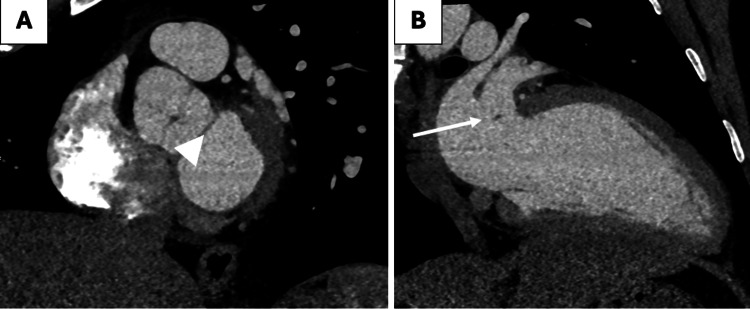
Cardiac CT in short axis (A) and two-chamber (B) planes post intervention showing a significant reduction in size of both the sessile (arrowhead) and mobile (arrow) post-catheter intervention with minimal residue.
